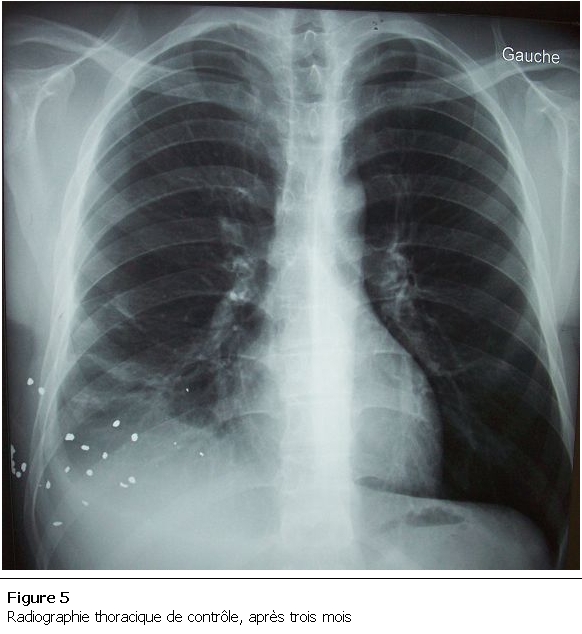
Radiographie thoracique de contrôle, après trois mois
